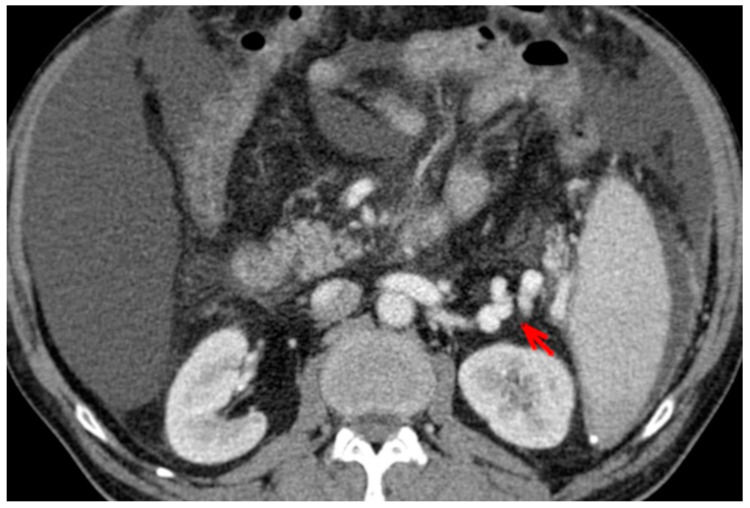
Axial contrast-enhanced CT shows spleno-renal shunt (red arrow).
